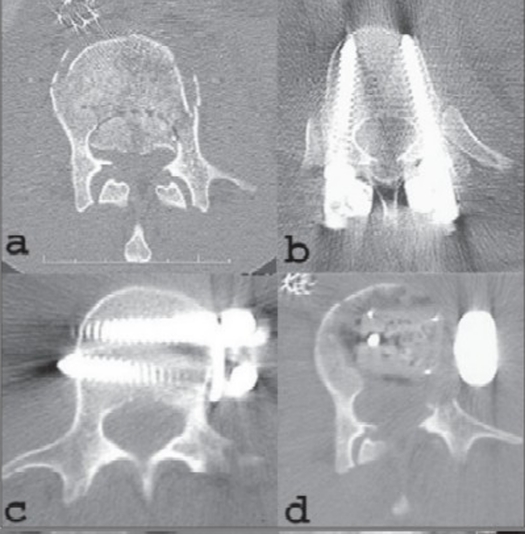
Axial CT scan (a) image at the level of the L2 pedicles demonstrates a 70% compromise of the spinal canal area by a large retropulsed fragment of the vertebra. Of note, the L1 vertebra had a 55% loss of spinal canal area. The patient was operated for -AP spinal reconstruction: Anterior surgery includes corpectomies of L1 and L2, placement of a stackable carbon fiber cage filled with autograft bone anteriorly from T12 - L3 and stabilization with a Kaneda screw-rod construct. Posteriorly the stabilization with bilateral pedicle screws at T11 and L4, bilateral hooks at T12 and L3, two rods with two crossconnectors and generous amounts of autologous iliac crest bone graft was done. (b) Axial CT scan at the level of the T11 pedicles demonstrates well-positioned pedicle screws which approach the far bony cortex of the T11 vertebra. Axial CT scan (c) at the level of the L3 pedicles demonstrates the inferior Kaneda screws which are placed across the vertebra to achieve bicortical fixation. Axial CT scan (d) through the L2 level which shows the pedicle-to-pedicle decompression of the spinal canal with the carbon fiber cage filled with autograft
